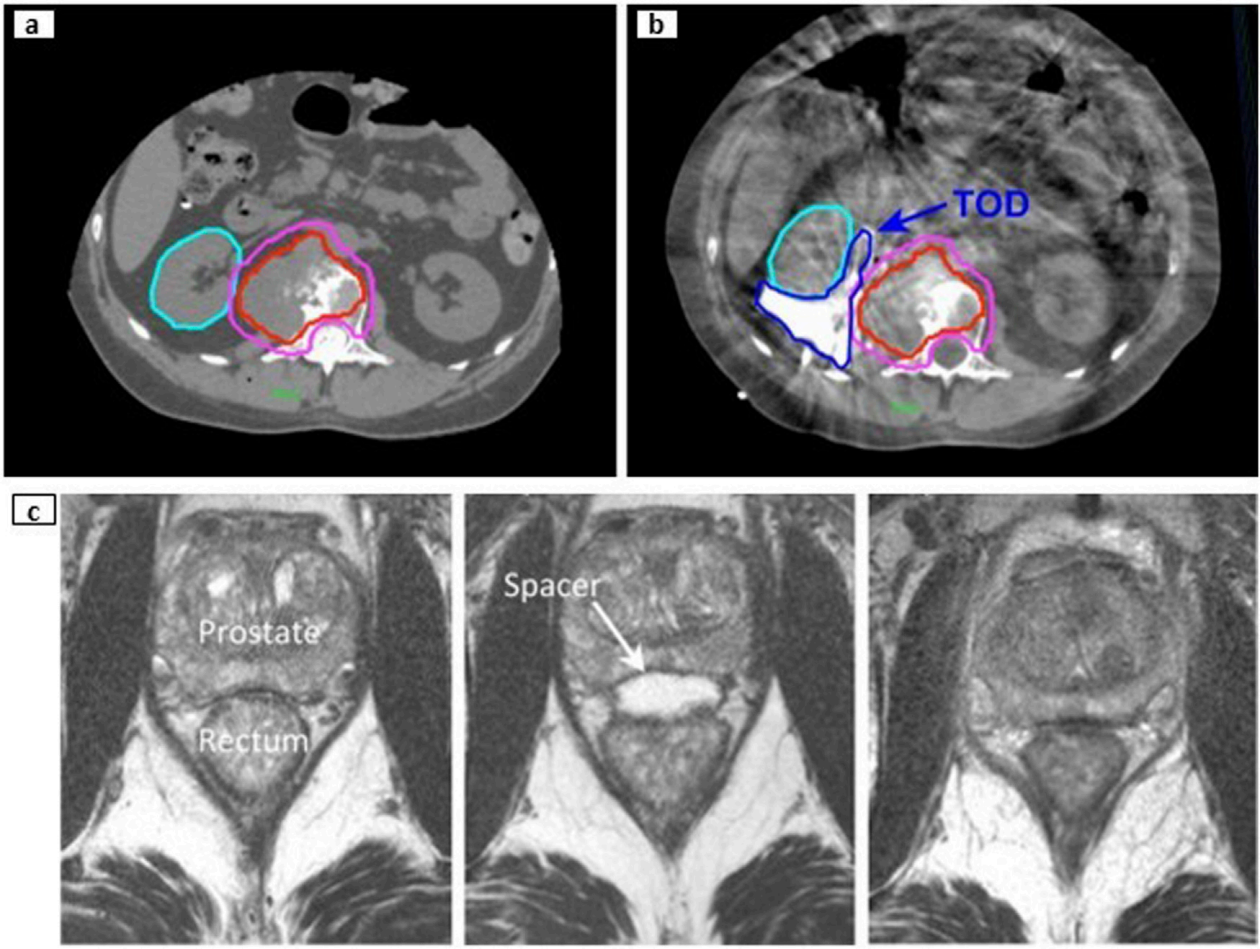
Examples of injectable spacers. (a) Baseline CT scan of a sacral chordoma, highlighting the proximity of the pelvic sacral tumor to the bowel at the S2-4 vertebrae. (b) Cone-beam CT image demonstrating successful temporary organ displacement (TOD) of the bowel, achieved through the injection of sterile saline via hydrodissection, creating a protective margin between the bowel and the tumor target. (a) and (b) Reproduced with permission from “Axial imaging of paraspinal non-seminomatous germ cell tumor treated to 2850 cGy” by Evangelia Katsoulakis, Stephen B Solomon, Majid Maybody, Douglas Housman, Greg Niyazov, Nadeem Riaz, Michael Lovelock, Daniel E Spratt, Joseph P Erinjeri, Raymond H Thornton and Yoshiya Yamada, licensed under CC BY 2.0. (c) Visualization of the TOD space between the prostate and rectum using SpaceOAR™ across varying timepoints. The SpacerOAR™ is a polyethylene glycol hydrogel material designed to spare the rectum during RT. Reproduced with permission from “T2-weighted magnetic resonance images of a spacer patient at baseline (a), post-application (b), and 12 months after spacer application (c)” by Mariados et al., licensed under CC-BY-NC-ND.
